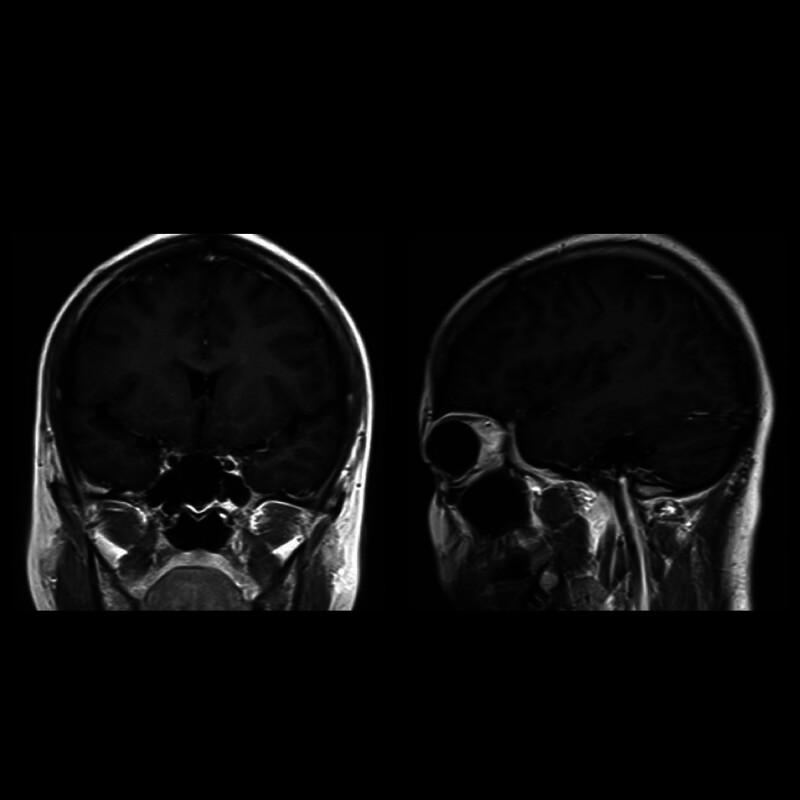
MRI showed fewer signal abnormalities and no new lesions after treatment. MRI = magnetic resonance imaging.
